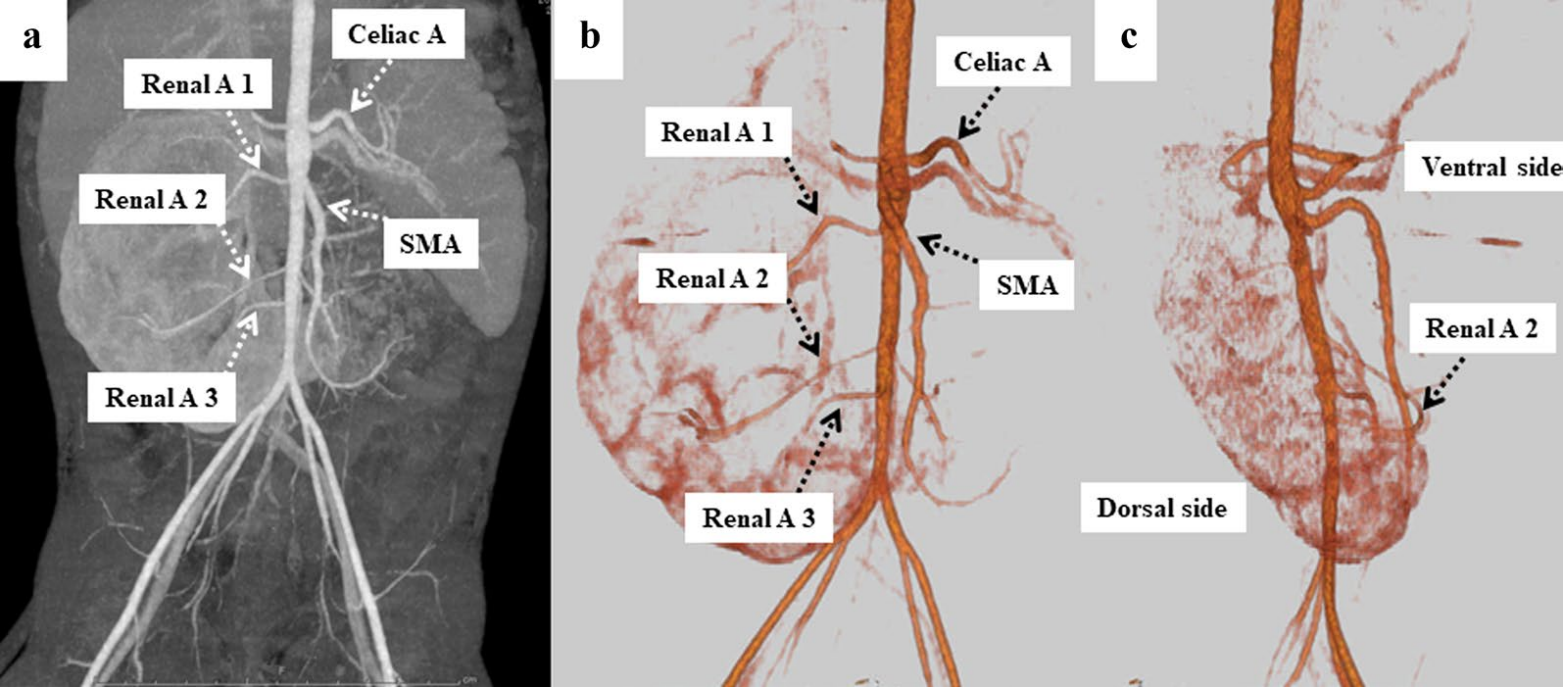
Preoperative enhanced CT findings
